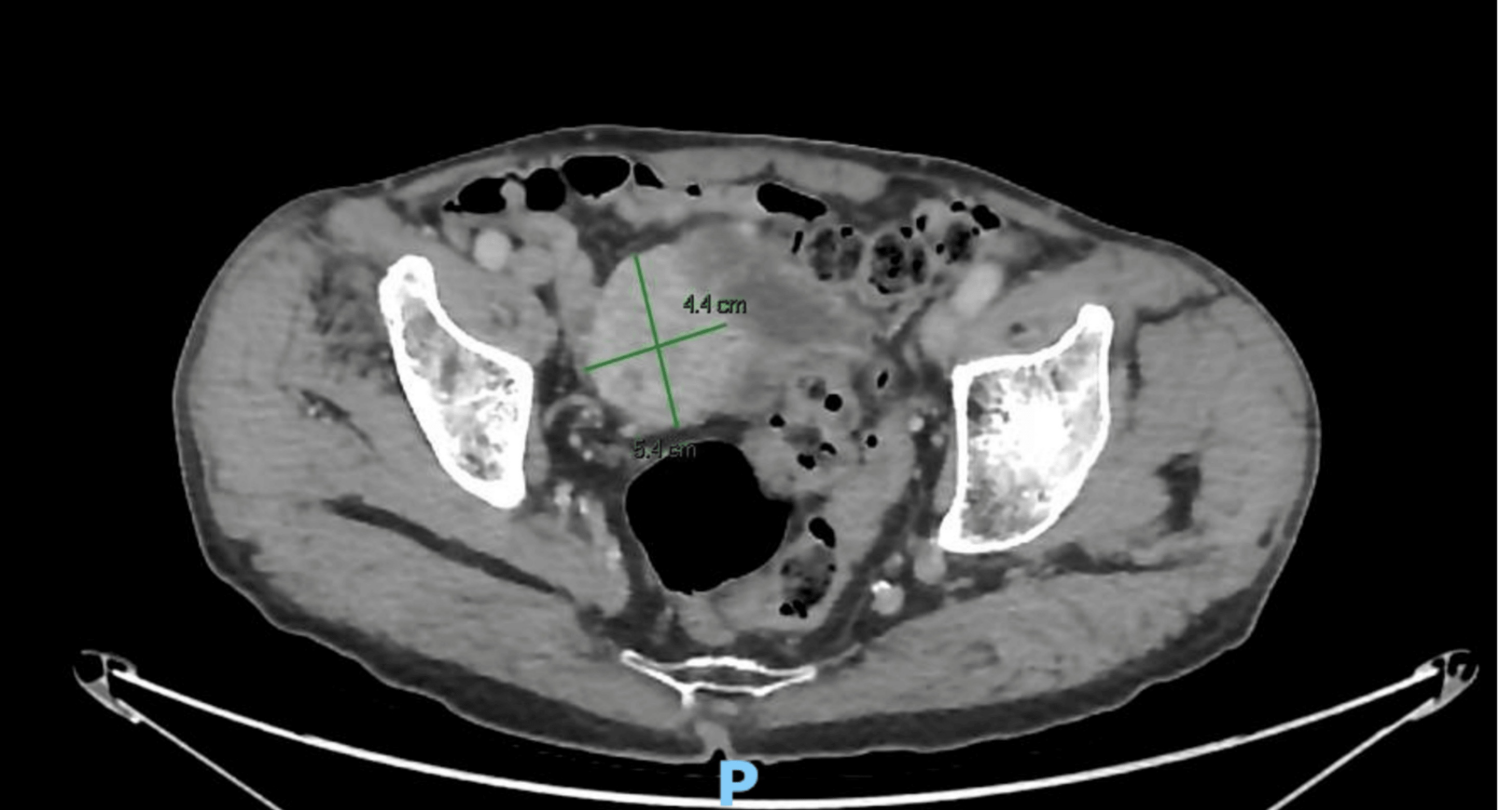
Contrast-enhanced CT imaging with a solid lesion of 5.4×4.4 cm on the right posterolateral wall of the bladder, with irregular margins
